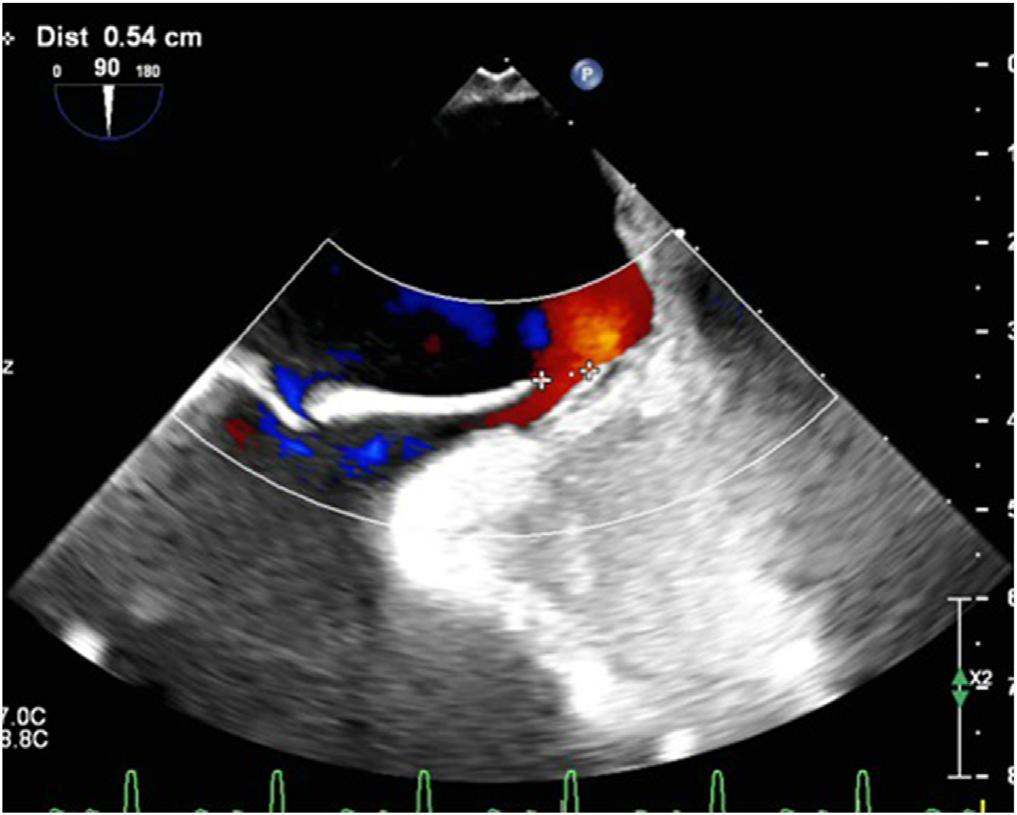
Transesophageal echocardiogram on postoperative day 3 showing the long-tunnel patent foramen ovale with significant interatrial flow.
